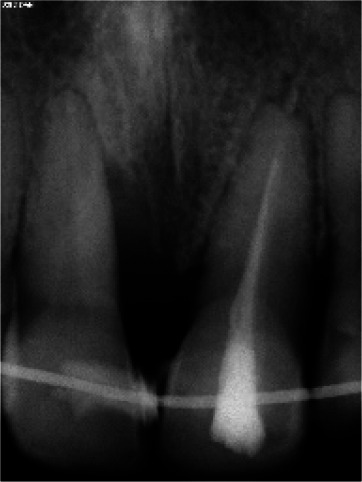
A vertical bone destruction extending to the third part of the root at the mesial site of the right incisor is visible on a peri-apical radiograph
